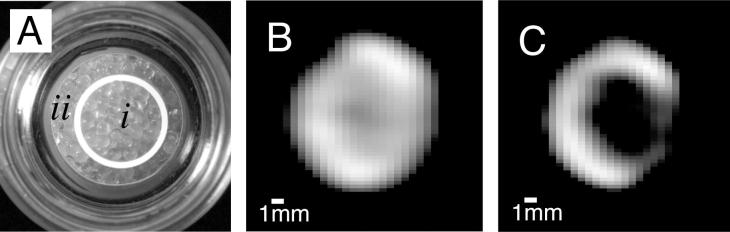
(A) Photograph of a sample containing 1.0 mm glass beads with a siliconized, hydrophobic surface in compartment (*i*) and an untreated, hydrophilic surface in compartment (ii). (B) MRI of gas phase hp ^83^Kr shortly after transfer into the sample. (C) Hp ^83^Kr MRI as in (B) but after additional 6 s delay time, showing a surface sensitive MRI contrast. The ^83^Kr quadrupolar relaxation caused by surface interactions leads to *T*_1_ = 9 s in the hydrophobic region (i) and to *T*_1_ = 35 s in the hydrophilic region (ii). Adapted figure, printed with permission from Pavlovskaya et al. P. Ntl. Acad. Sci. USA 2005; 102:18278 © 2005 National Academy of Sciences, USA.
